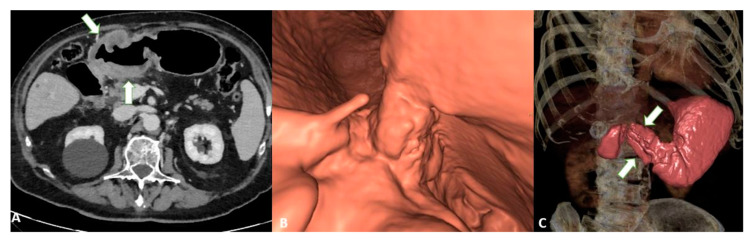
T4a gastric cancer in a 53-year-old female patient. (A) Axial 2D image in the portal venous phase with distension of the gastric lumen with air shows a bulky circumferential tumor (white arrows) of the low body of the stomach and of the gastric antrum with ulcerations; (B) Virtual gastroscopy delineates a lesion protruding in the lumen of the stomach; (C) Computed tomography gastrography shows a mucosal irregularity (white arrows) with a reduction of the lumen of the stomach.
